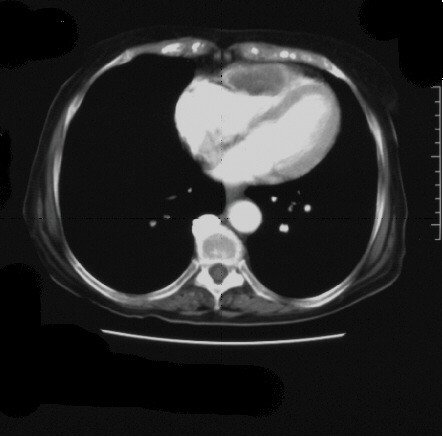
Contrast CT thorax demonstrating filling defect and lesion in right anterior ventricular wall.
